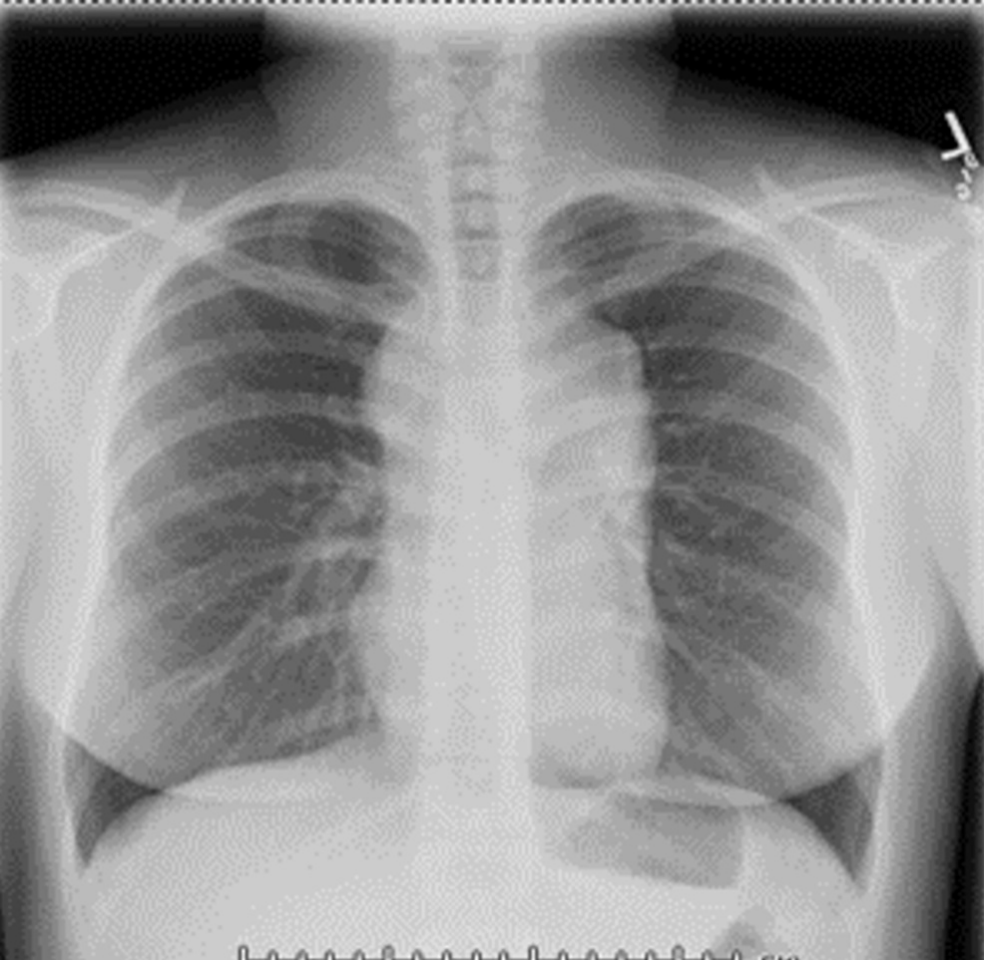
Initial Chest X-ray Revealed Large Mediastinal Adenopathy
